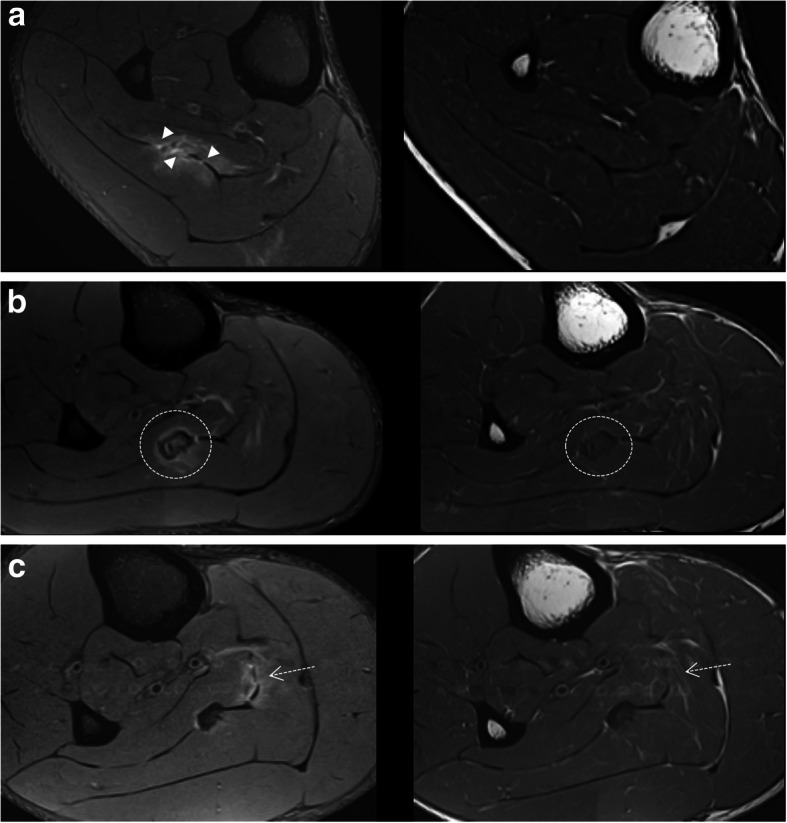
Evolution of healing by MRI over a course of 8 weeks: soleus with common aponeurosis, a normal anatomical variant, myoconnective junction. Axial T1- and fluid-sensitive images at 24 h (a), 4 weeks (b), and 8 weeks (c). a MR images show an extensive initial lesion with several foci of rupture of the lateral portion of the common aponeurosis (arrowheads). b MR images show an hypertrophic soft scar (dashed circle). c MR images show a medial re-injury in the common aponeurosis (dashed arrow)
